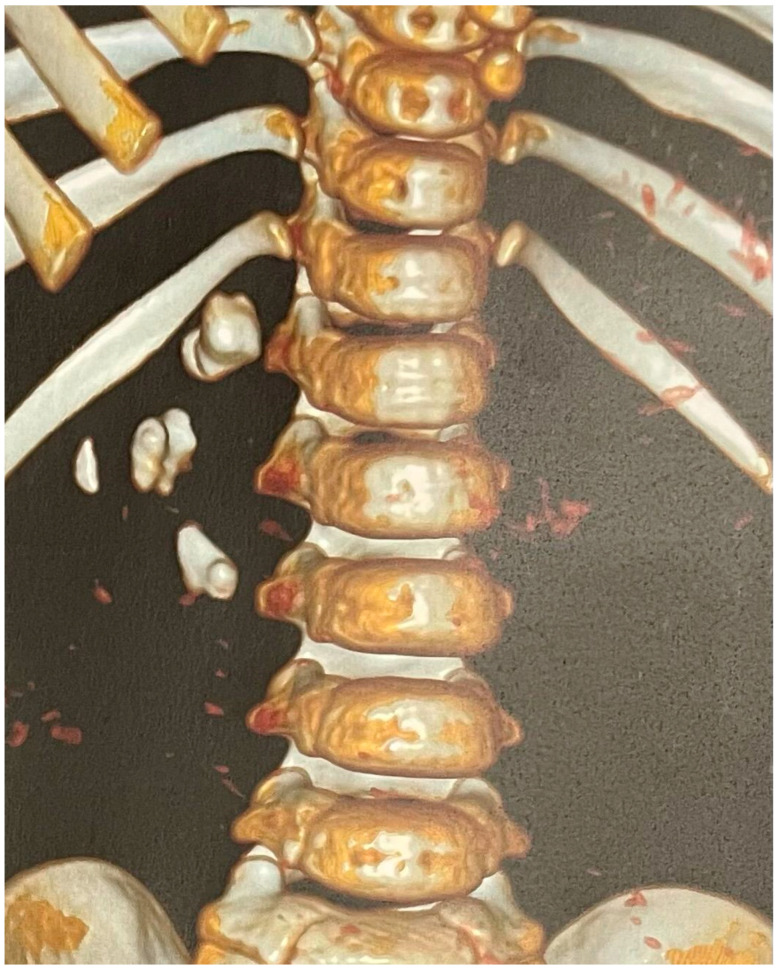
The 3D reconstruction of the preoperative CT scan of this case showing the stones in the right kidney.
